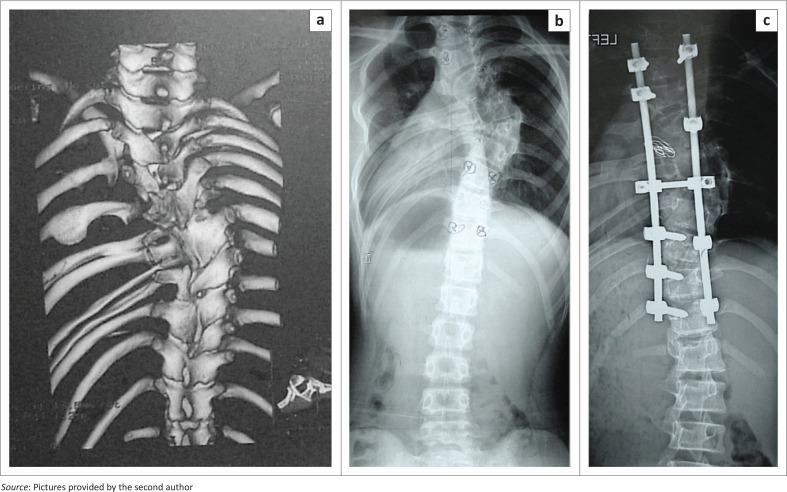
(a) CT reconstruction of the spine and ribs in a patient with congenital scoliosis including vertebral malformations and a segmentation disorder (rib synostosis). *(b)* Native x-ray, and (c) Spine after dorsal double rod instrumentation with pedicle screw fixation.
